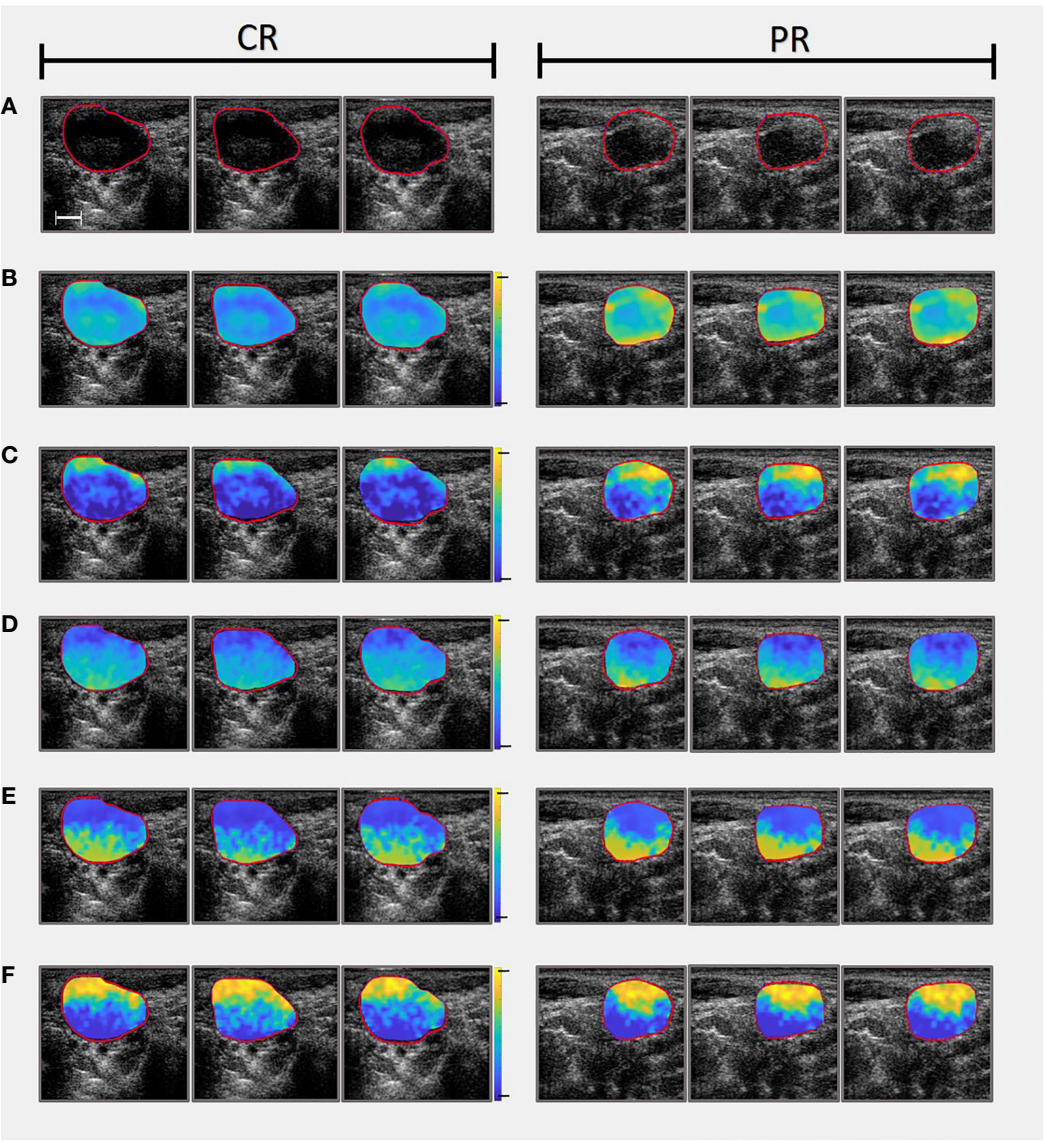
Comparing parametric maps (used as images to determine textural features from) and US slices of one CR patient (On the left) and one PR patient (On the right). (A) Presents the B-mode ultrasounds and accompanying LN ROIs. (B) Mid-Band Fit parametric maps (range from -10 to 40 dB). (C) Spectral Intercept parametric maps (range from 10 to 50 dB). (D) Spectral Slope parametric maps (range from -5 to 5 dB/MHz). (E) Average Acoustic Concentration calculated using the Gaussian model (range from 20 to 220 dB/cm^3^). Note that Average Acoustic Concentration was also calculated using Anderson model but omitted from this figure for convenience). (F) Average Scatterer Diameter calculated using the Gaussian model (range from 0 to 150 µm). Note that Average Scatterer Diameter was also calculated using Anderson model but omitted from this figure for convenience). Scale bar is 5 mm.
